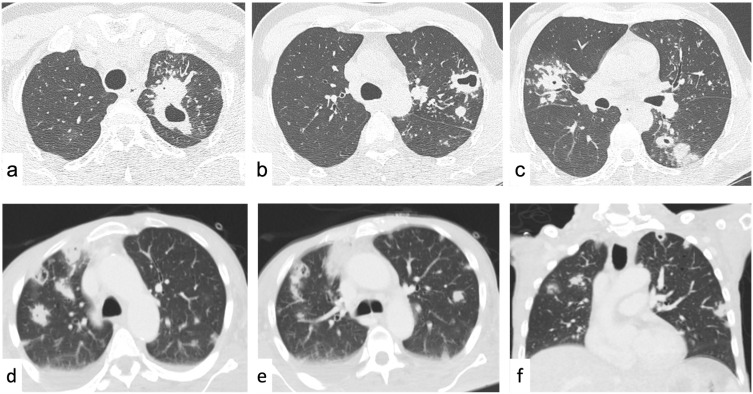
Excavated nodules vs. septic emboli. Axial CT scans (lung window) in a 48-year-old male patient with asthenia, fever, and productive cough for several days (a–c). It shows multiple solid nodules and micronodules with centrilobular distribution, with a “tree-in-bud” pattern, larger nodules, and some excavated lesions at the upper lobes and apices (tuberculosis). Axial CT scans (d,e) with coronal reconstruction (f) in a 54-year-old male patient presenting with fever and laboratory evidence of septic shock caused by the presence of infective endocarditis from Staphilococcus Aureus. Solid nodules, some with cavitation and peripheral ground glass, joined with the clinical data suggested the diagnosis of septic emboli.
